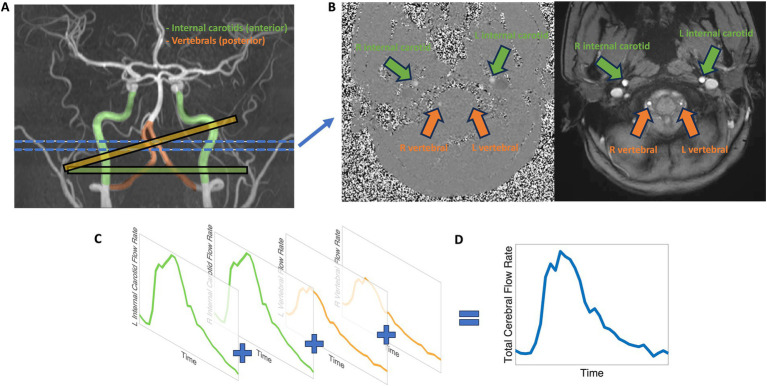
Visualization of internal carotid and vertebral artery flow measurements, representing anterior (green) and posterior (orange) cerebral blood flow, by phase contrast MRI. (A) The internal carotid arteries (green), representing the anterior circulation, and the vertebral arteries (orange), representing the posterior circulation are measured using phase contrast. Example 2D phase contrast plane perpendicular alignments are shown for the internal carotid arteries (green plane) and vertebral arteries (orange plane). The blue lines indicate the approximate target location for blood vessel measurement. (B) 2D phase contrast MRI produces cardiac-gated magnitude (right) and phase (left) cross-sectional slices. (C) Representation of typical internal carotid and vertebral blood flow profiles. (D) The sum of internal carotid and vertebral blood flow profiles characterizes extracranial total cerebral blood flow.
